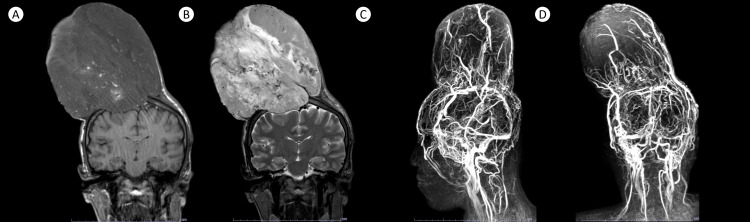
Representative images of the patient’s MRI show a large heterogeneously enhancing tumor, measuring 14.5x14.2x11.9 cm (APxTxCC), shown in coronal T1-weighted (A), T2-weighted (B), sagittal (C), and coronal (D) images. MRV images show a highly vascular tumor that receives supply from scalp arteries and drains via cortical and emissary veins.
